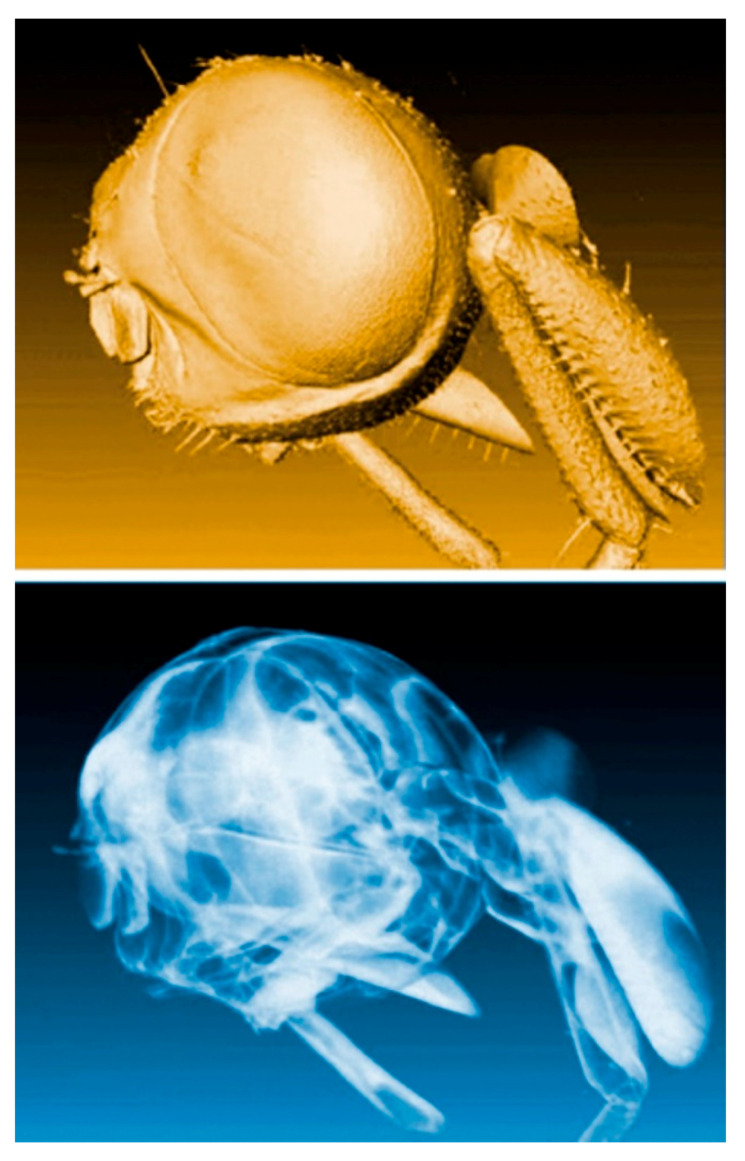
Two other coherence-based X-ray images of part of a housefly [14].
